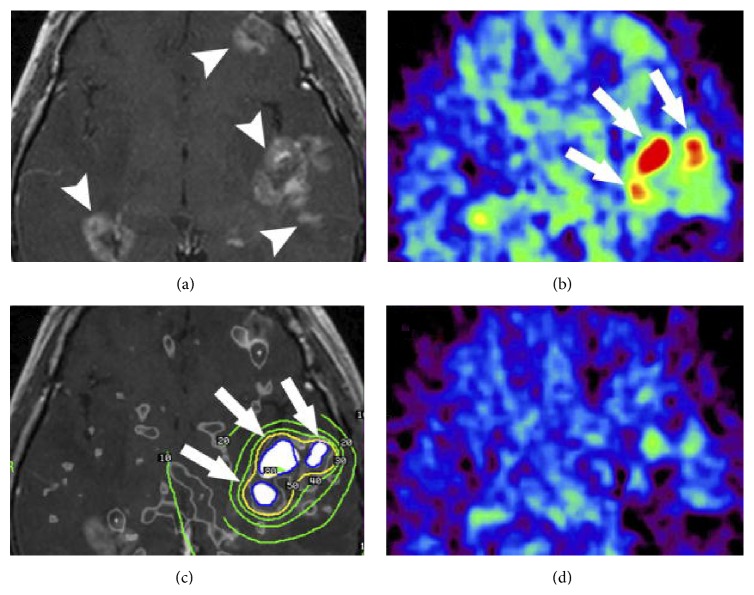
Representative plan for GK surgery treating locally recurred brain metastases with guidance by ^11^C methionine- (MET-) PET/MRI fusion imaging. The patient was a 42-year-old woman with brain metastases from breast cancer who had received 4 previous treatments with GK. (a) A big discrepancy was clearly seen between the area with good contrast enhancement on the T1-weighted MR image (indicated by arrowheads) and (b) the area with elevated MET uptake (indicated by arrows). (c) The PET-MRI fusion image was rendered after constructing a binary image (shown in white), indicating areas with MET uptake 1.4 times higher than that in the contralateral brain. (d) The GK strategy was to administer a higher radiation dose to areas with higher MET uptake. ^11^C methionine uptake was markedly reduced in a MET-PET image obtained 6 months after the GK (i.e., good control of active cancer). Reprinted, with permission, from [40].
